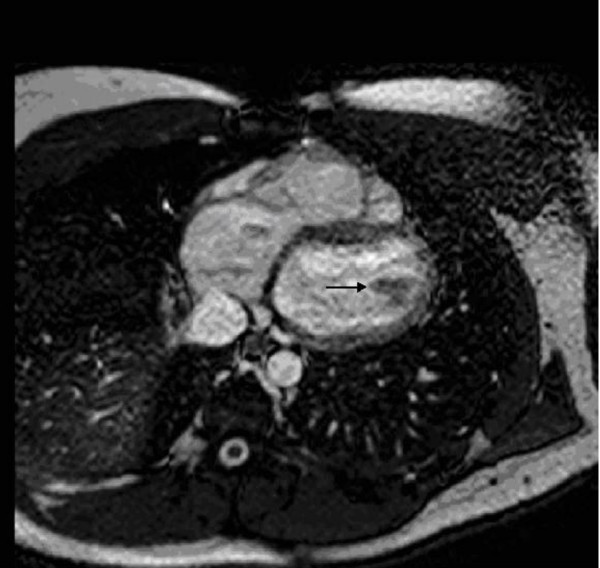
Cardiac MRI: T1WI, with contrast, demonstrates low intensity mass presenting the myxoma.
